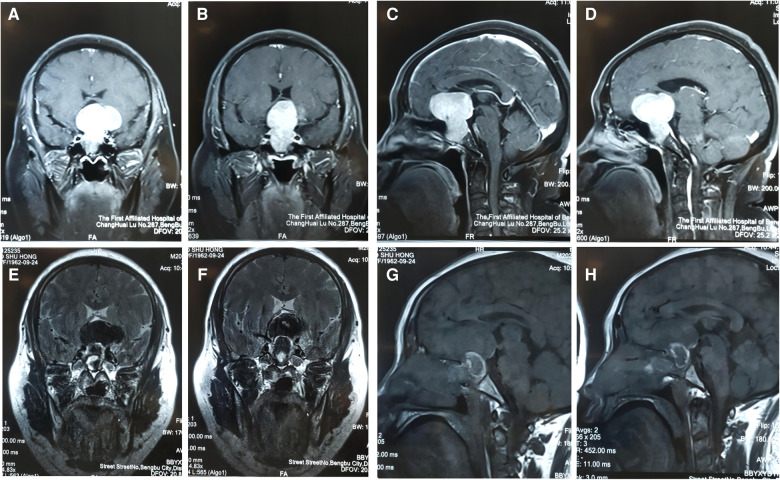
(A–H): This was a 50-year-old female patient who was admitted to hospital after the discovery of a pituitary tumor due to clinical manifestation. Preoperative MRI of a special giant pituitary adenoma (A–D). Postoperative MRI (E–H).
